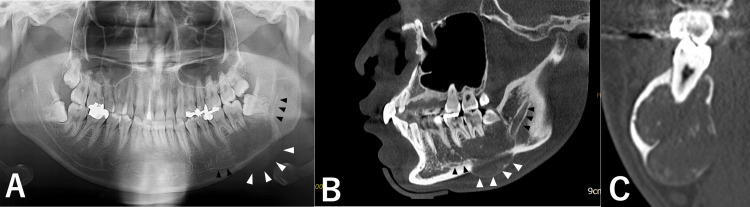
Panoramic and CBCT findings A: Panoramic image. Panoramic radiograph shows an expansile lesion extending beyond the inferior border of the mandible (white arrowheads), with inferior displacement of the mandibular canal (black arrowheads). B, C: CBCT image. CBCT shows expansile lesion with mixed densities and partially ill-defined borders, extending beyond the mandibular border (white arrowheads), displacing the mandibular canal inferiorly (black arrowheads), and partially encasing the neurovascular bundle (C). CBCT: Cone-beam CT
